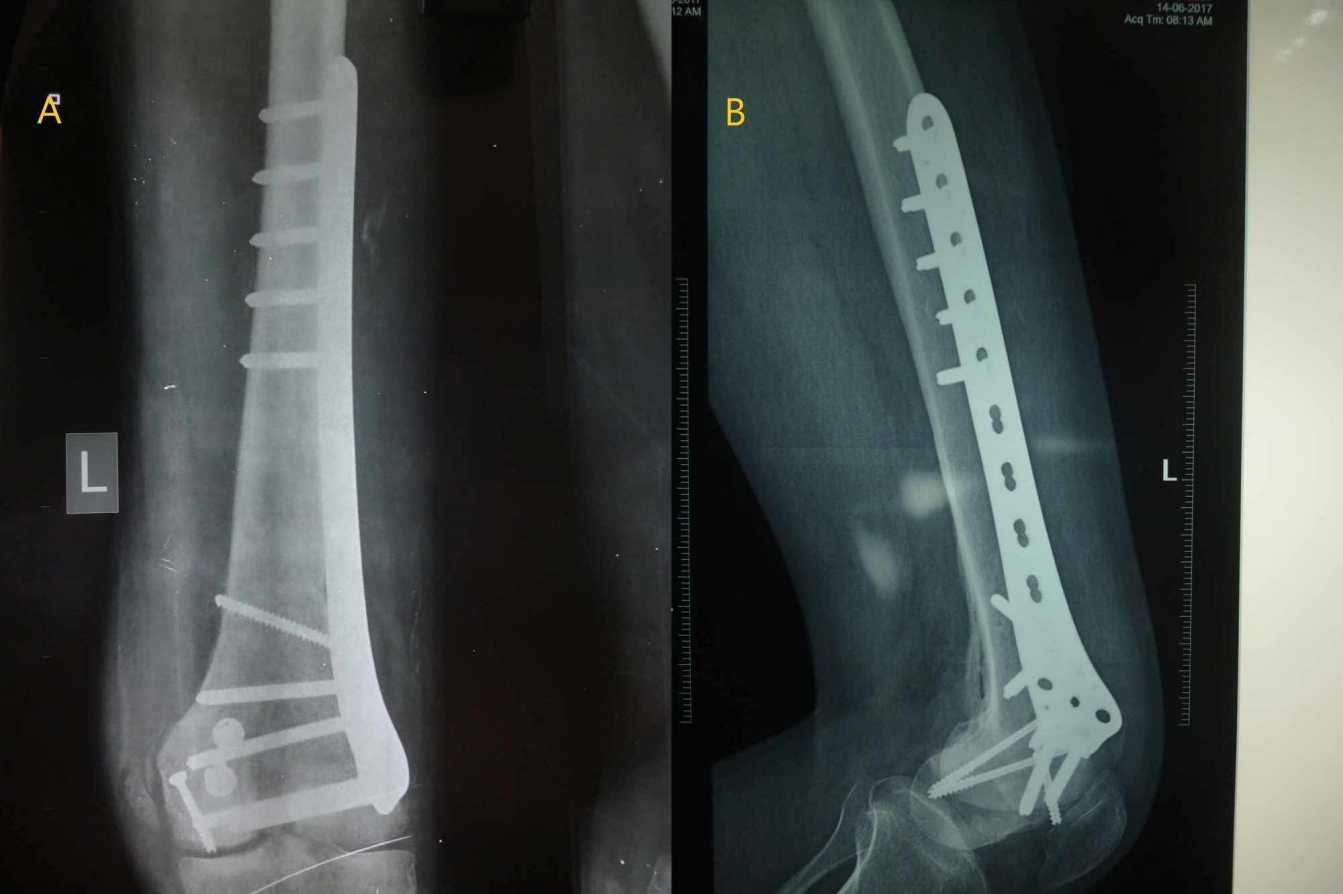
Radiographs of the knee at the final follow-up A: anteroposterior view; B: lateral view. The images show complete bony union
